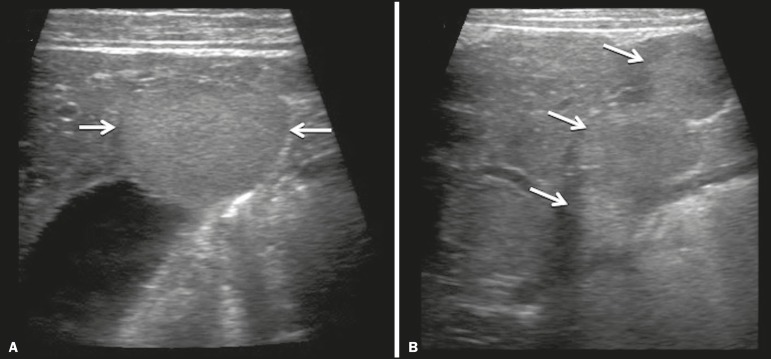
Multifocal nodular subcapsular hepatic steatosis in an 8-year-old boy with chronic liver disease and cystic fibrosis. Follow-up ultrasound showing diffuse heterogeneity of the liver parenchyma and subcapsular hyperechoic multifocal lesions, some with a nodular appearance (arrows), without causing a mass effect.
